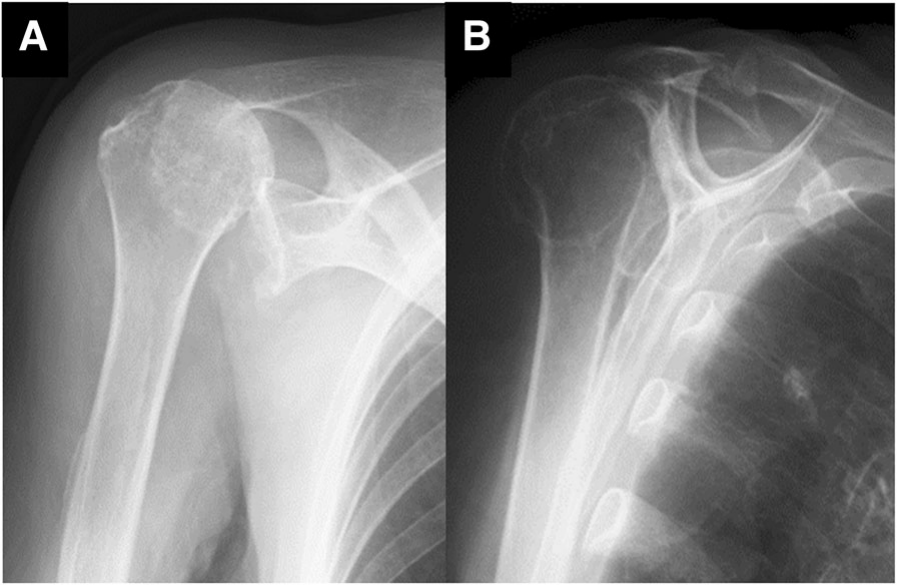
X-ray radiography images soon after the removal of orthosis revealing re-dislocation (a, b)
